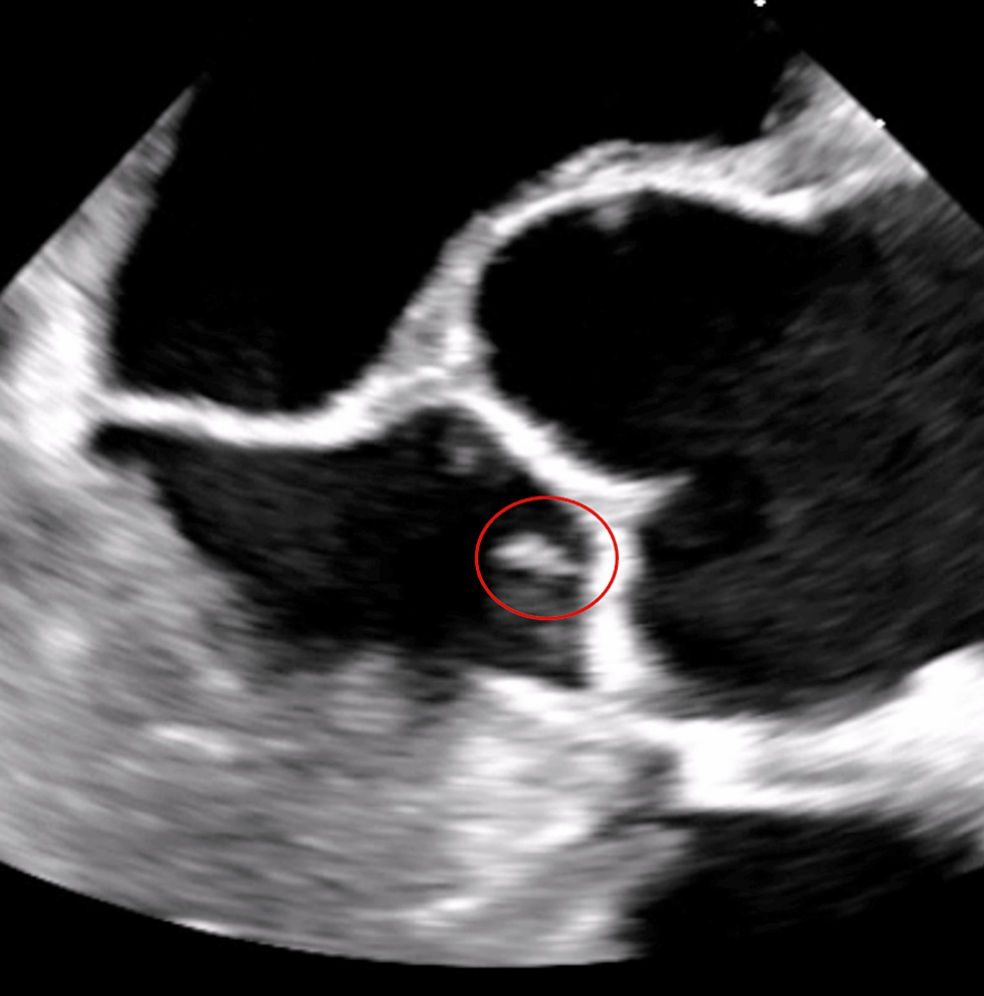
Transesophageal echocardiography showing sclerotic aortic valve and an echogenic, highly mobile structure (red circle) attached to the ventricular surface of the right coronary aortic valve cusp measuring 0.8 x 0.25 cm.
